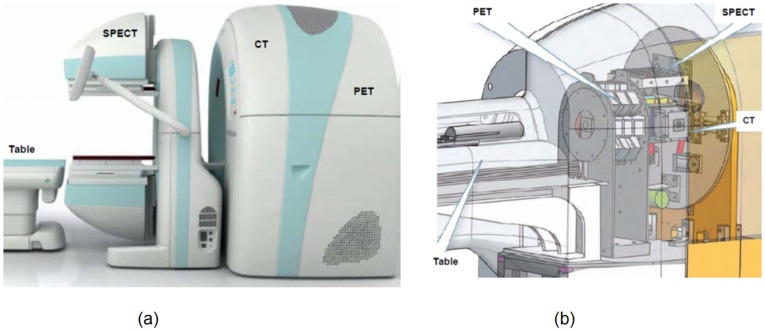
State-of-the-art tri-modality fusion systems. (a) The AnyScan system for clinical PET-SPECT-CT, and (b) the Albira system for preclinical PET-SPECT-CT ((a) and (b) from http://www.mediso.de/anyscan-sc.html and http://www.cmi-marketing.com/7modalities respectively, with the legends added by the authors of this article).
